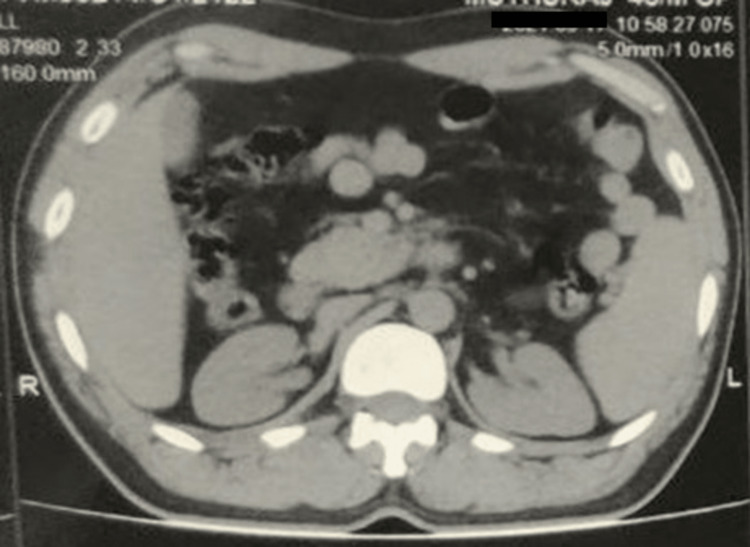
Computed tomography shows dorsal agenesis in a patient with acute mild pancreatitis
